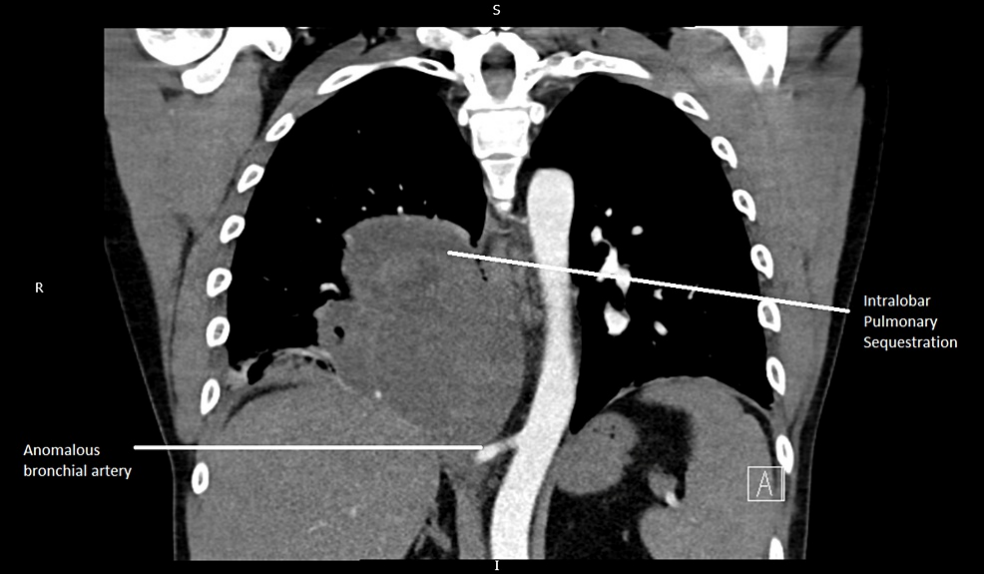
CTPA coronal section (image from the admission scan showing IPS with anomalous bronchial artery) CTPA: CT Pulmonary Angiogram; IPS: Intralobar Pulmonary Sequestration.
